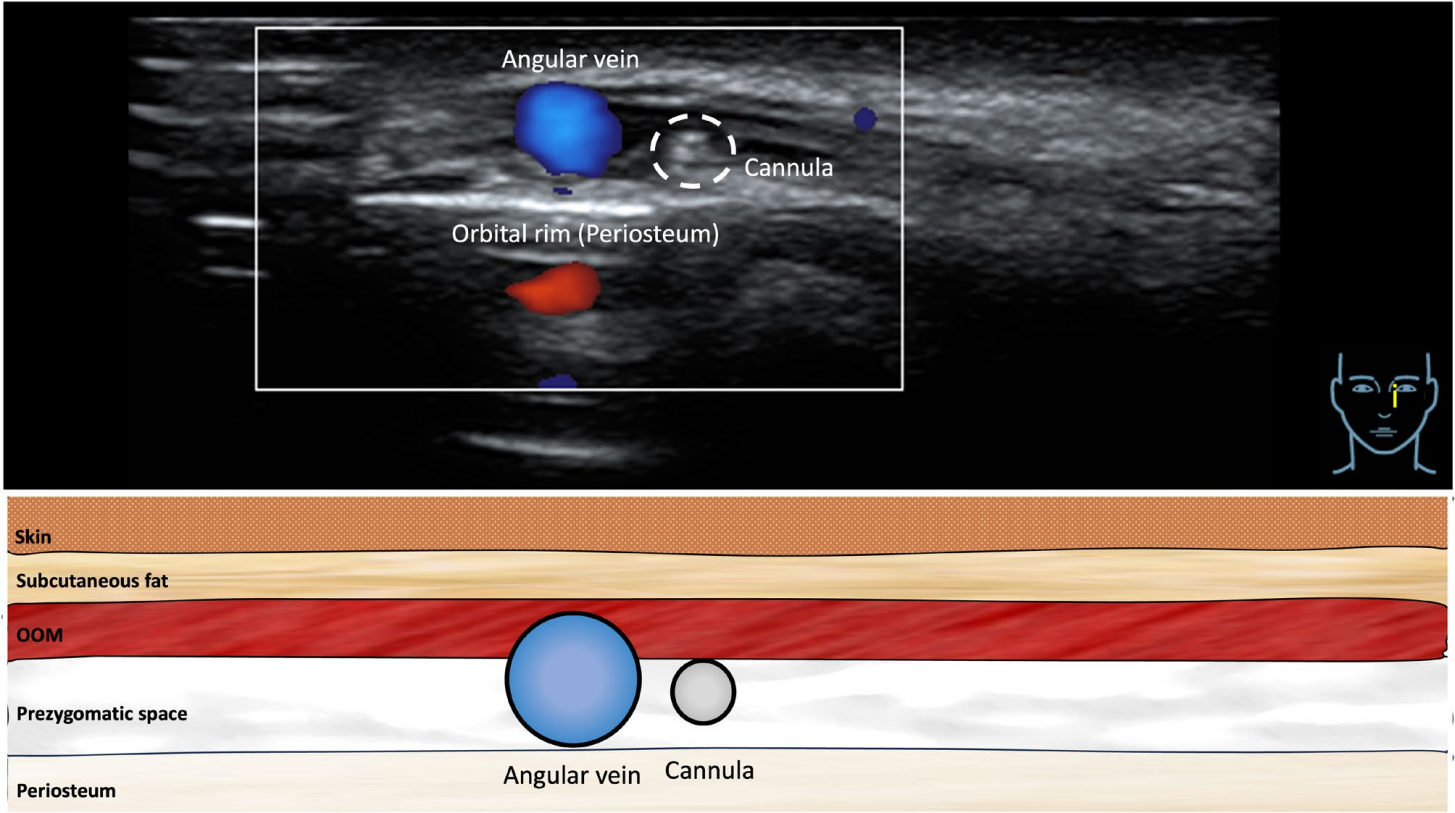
Ultrasound image showing the location of the cannula in the prezygomatic space right next to the angular vein and superficial to the orbital rim (= periosteum). The ultrasound transducer was placed in a vertical fashion.
